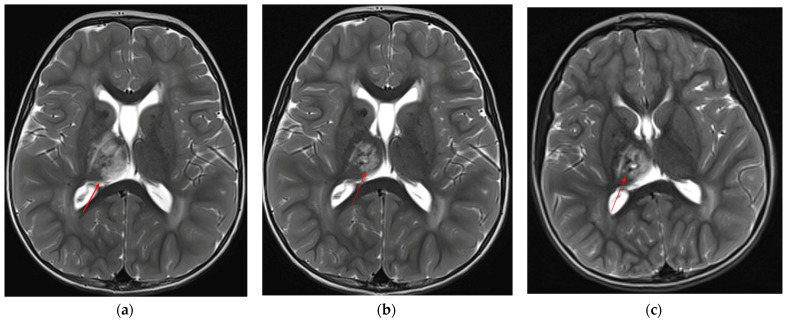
Axial T2-TSE showing progressive reduction and cystic degeneration of the right thalamic lesion (red arrows): (a) 2 years and 4 months of age, (b) almost 3 years, (c) last MRI at almost 5 years of age.
